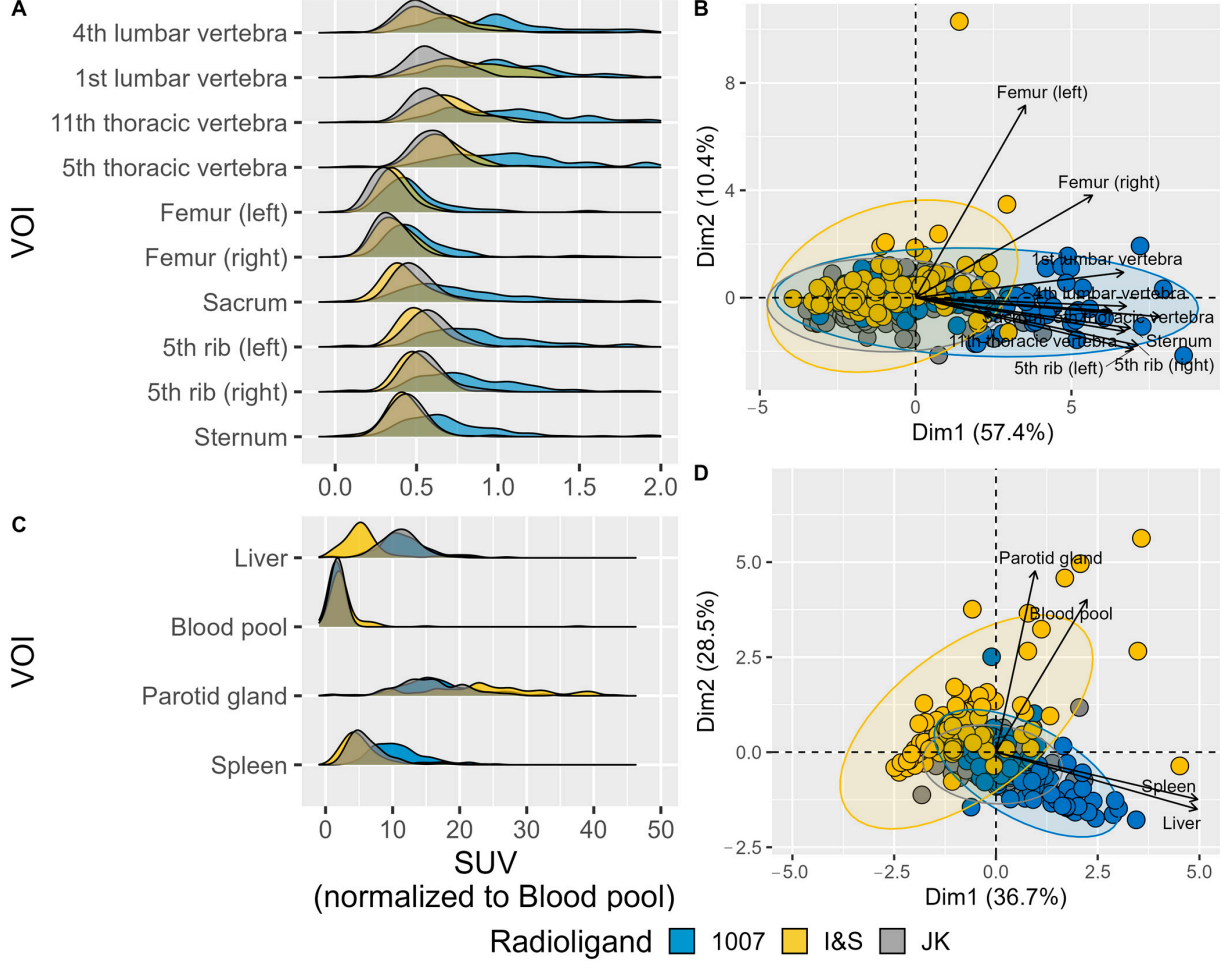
Normalized SUV peak values in different Volumes of Interest (VOIs). (A) Density plots showing the distribution of normalized SUV values for various bone VOIs. (B) PCA biplot visualizing the relationships among skeletal VOIs based on their peak SUV values. Different colors indicate the three radioligands, and arrows show the direction and magnitude of the VOI contributions to the principal components. The length of each vector represents the importance of the corresponding VOI in the PCA, with longer vectors indicating a greater contribution to the variance. The direction of the vectors indicates how each VOI is related to the principal components, with similar directions suggesting similar patterns of variation. (C) Density plots showing the distribution of peak SUV values (g/mL) for various reference VOIs. (D) PCA biplot visualizing the relationships among reference VOIs based on their peak SUV values. (The more readable (B) panel can be found as Figure A2).
